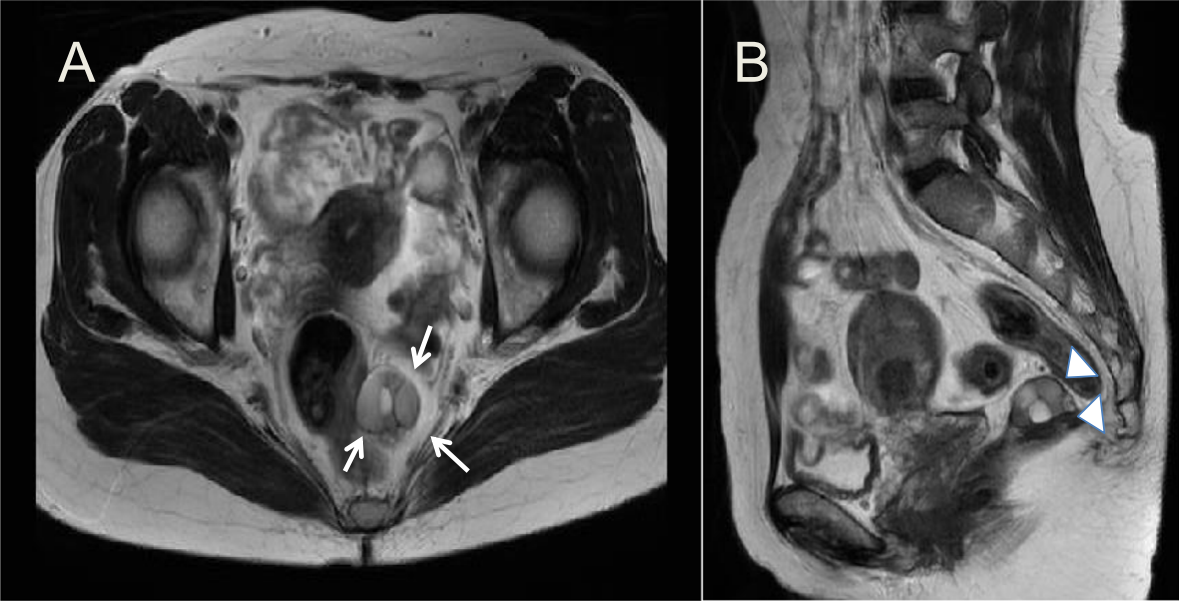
Magnetic resonance imaging. a On T2-weighted coronal magnetic resonance imaging, it appears that the 30-mm multicystic tumor on the left side of the posterior rectum is strongly adhered to the rectal wall (white arrow). b The enhancing cystic portions (white area in the center of the tumor) are accompanied by solid components (gray area at the margin of the tumor) (white arrowhead)
